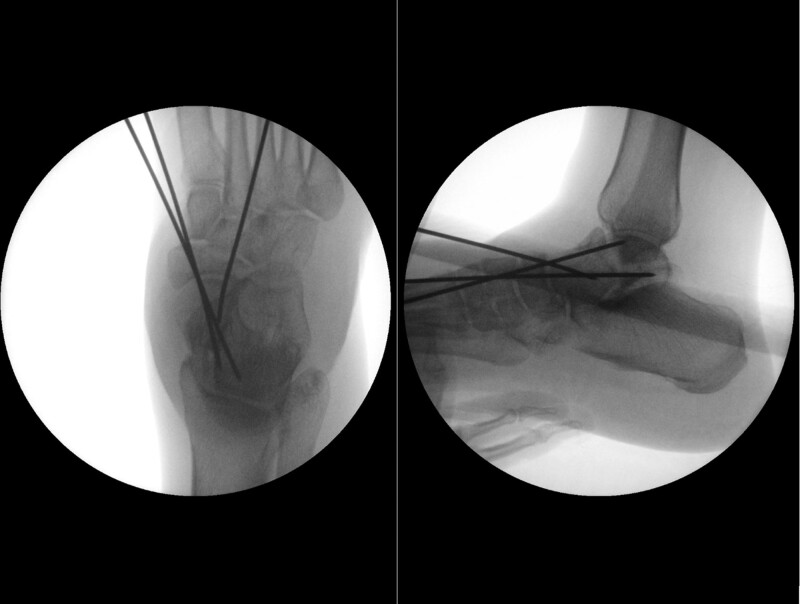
Intraoperative ankle X-rays. (A) AP and (B) LAT views confirming reduction of the talonavicular joint. AP = anteroposterior, LAT = lateral.
